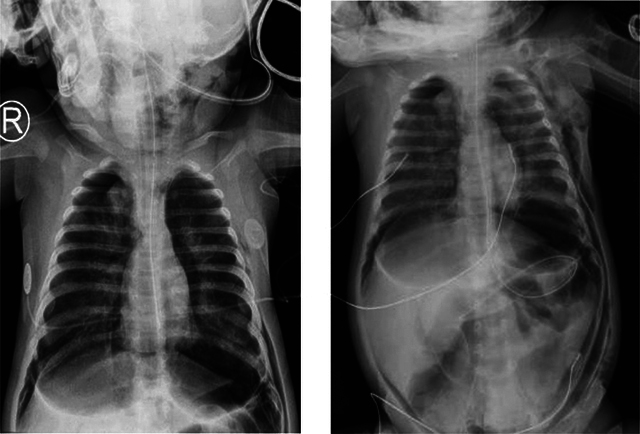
(A, B) Chest and abdominal antero-posterior radiograph. Free air (subcutaneous emphysema) located at the infra-thoracic part of the chest radiograph.
